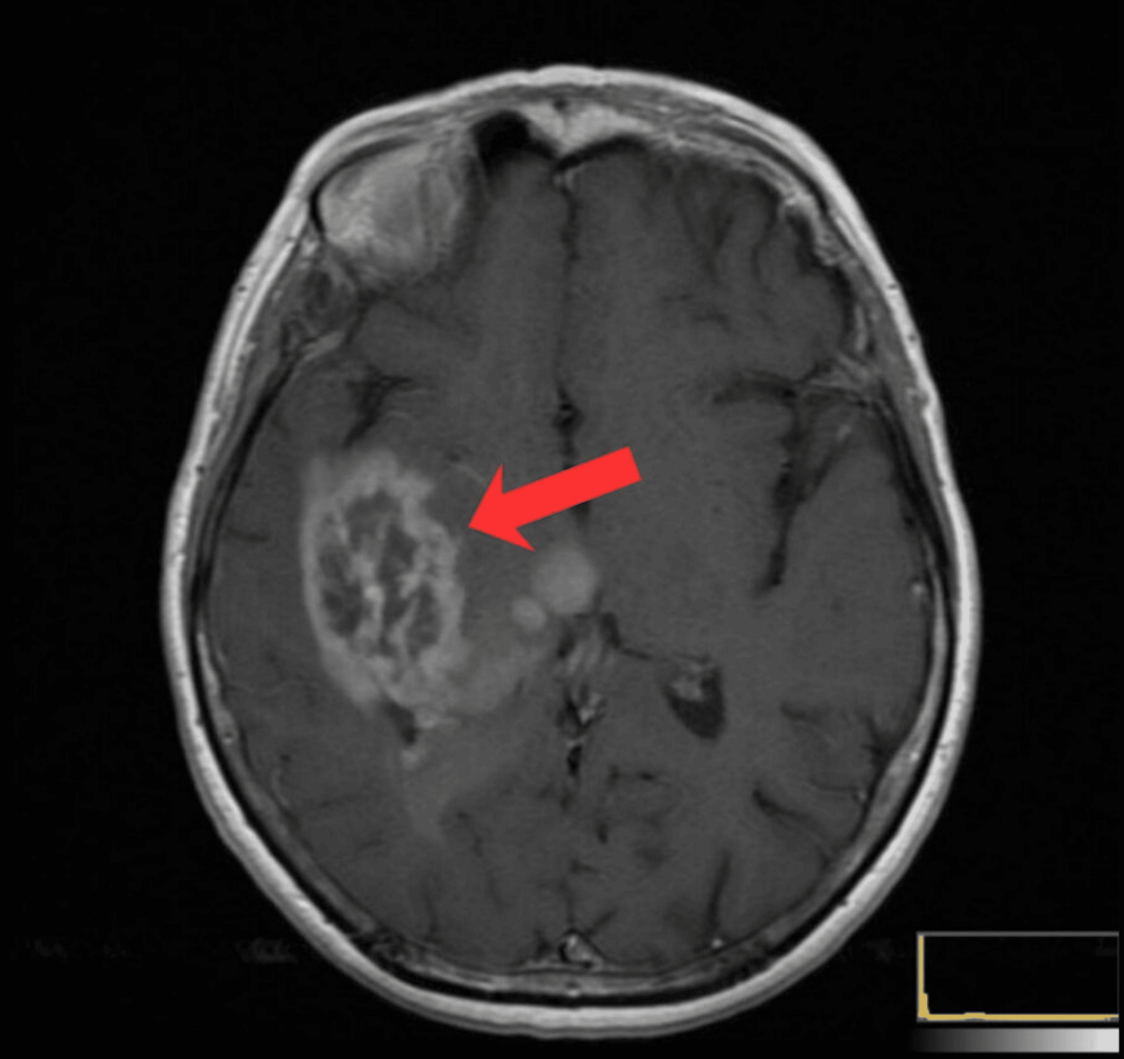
Axial contrast-enhanced MRI of a right temporal lobe mass Axial MRI demonstrating a large, heterogeneously enhancing mass in the right temporal lobe, characterized by a thick, irregular ring-enhancing rim and central necrosis.
